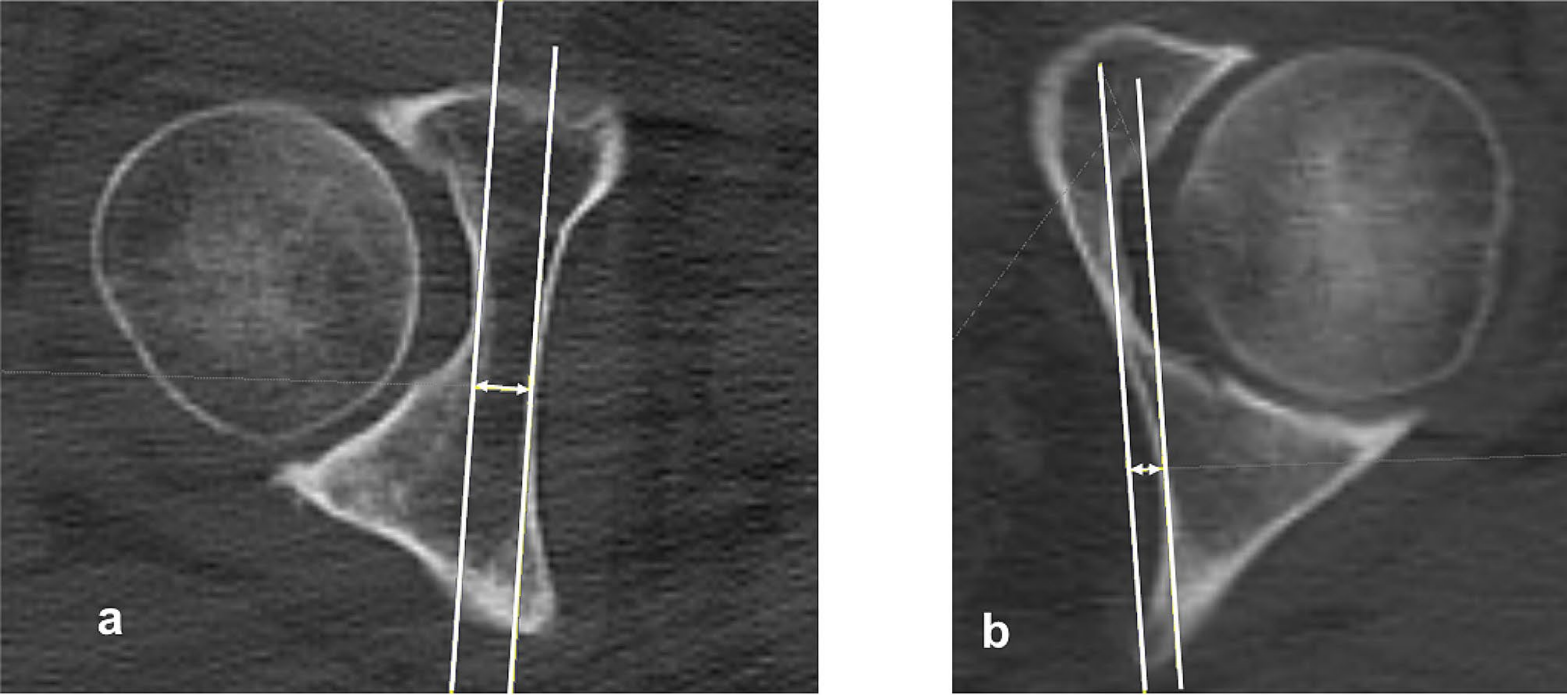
Measurement of the distance between line α and a line parallel to it and tangential to the acetabular floor. The bony region on axial CT images, which overlaps with the line (line α) with a perspective projection angle (inclined 4° to the vertical line) to the ilioischial line during radiography and tangential to the medial acetabular wall, represents the ilioischial line on the radiograph. The distance between line α and a line parallel to line α and tangential to the acetabular floor (white double arrow) was measured. a A coxa profunda-negative case. b A coxa profunda-positive case. CT, computed tomography
